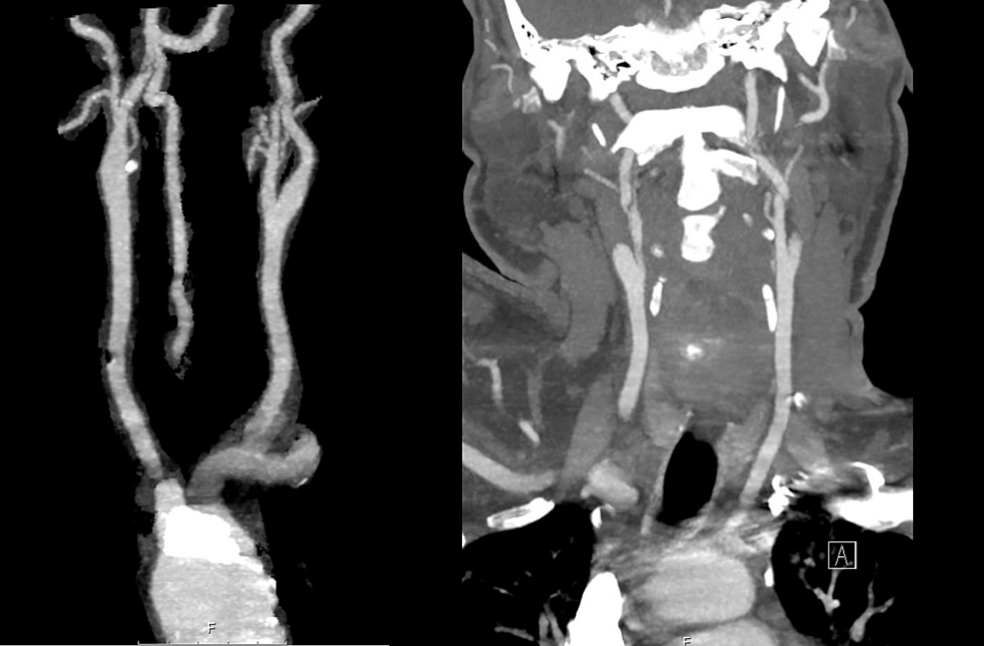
CT angiography of neck showing no evidence of hemodynamically significant carotid artery stenosis.
